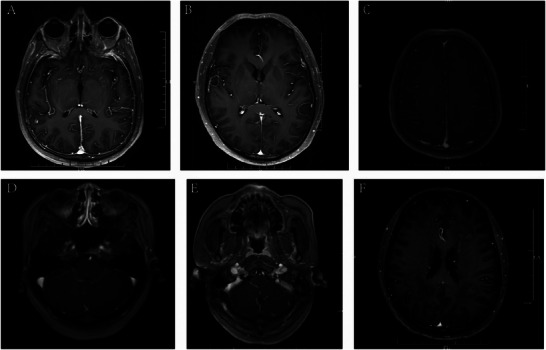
Enhanced cranial MRI shows enhancement of the dura mater, pia mater, paraventricular, and basal ganglia. (A) Significant enhancement of the dura mater; (B–C) Apparent enhancement of the pia mater in the cerebral hemisphere; (D–E) Apparent enhancement of the pia mater in the cerebellar hemisphere; and (F) Linear and patchy enhancement of the lateral ventricles bilaterally.
